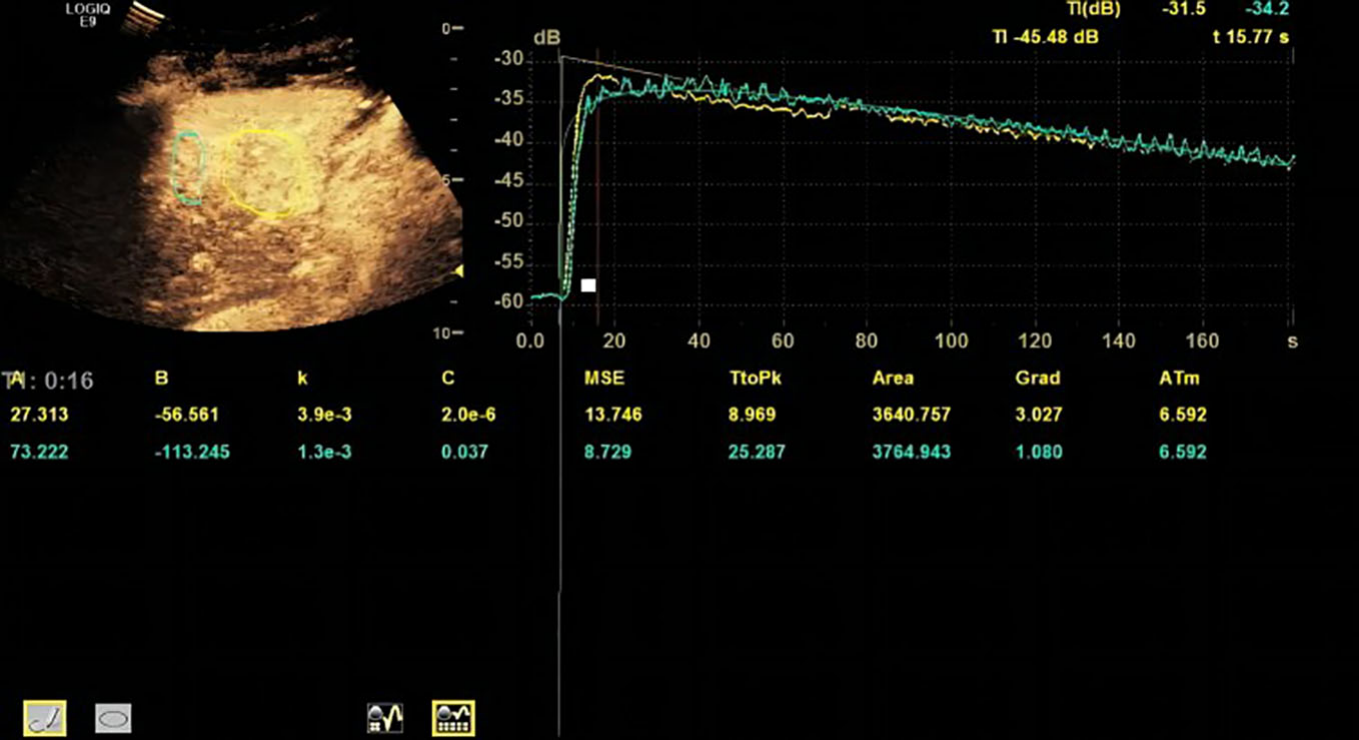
Representative image of contrast-enhanced ultrasound (CEUS) and time–intensity curve (TIC) of patients in the progressive disease (PD) group.
